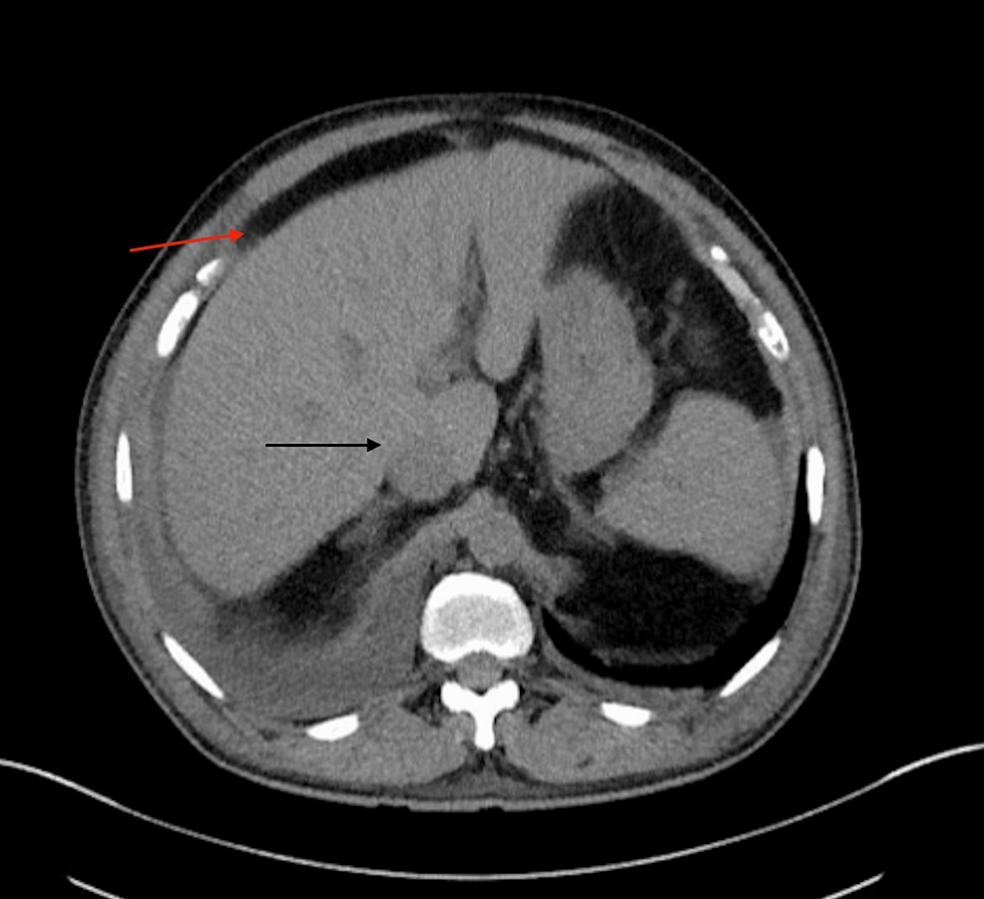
Computed tomography of the abdomen showing ascites (red arrow) and pericholecystic edema (black arrow)
